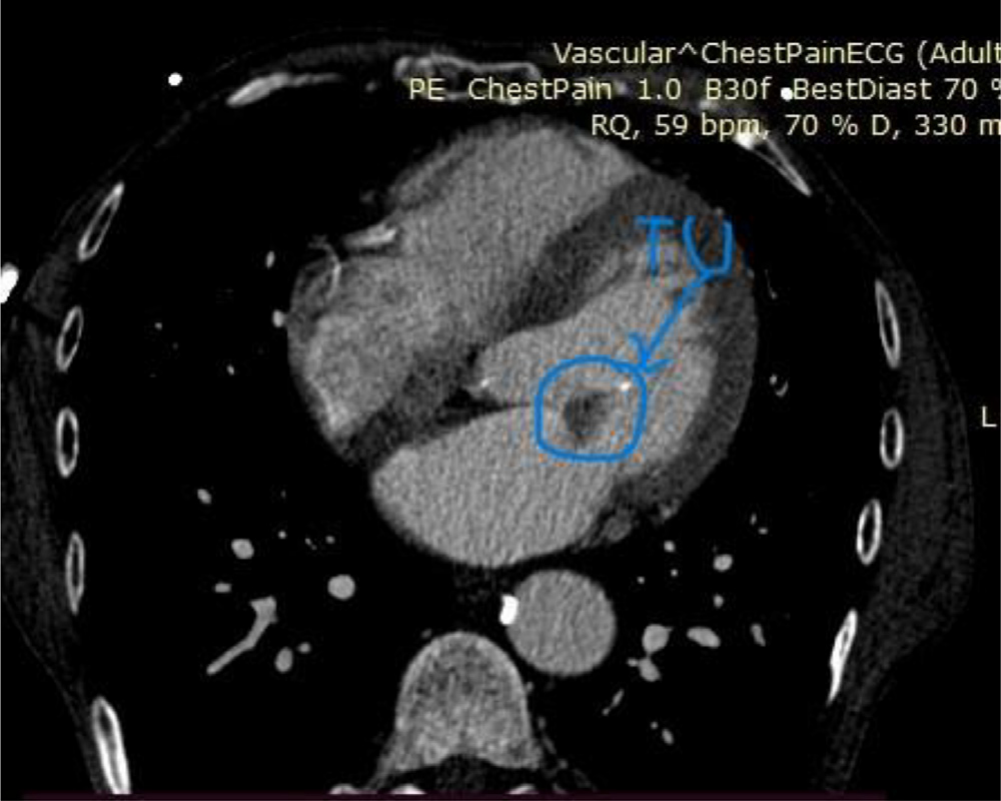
CT imaging of a tumorous mass at the mitral valve.
